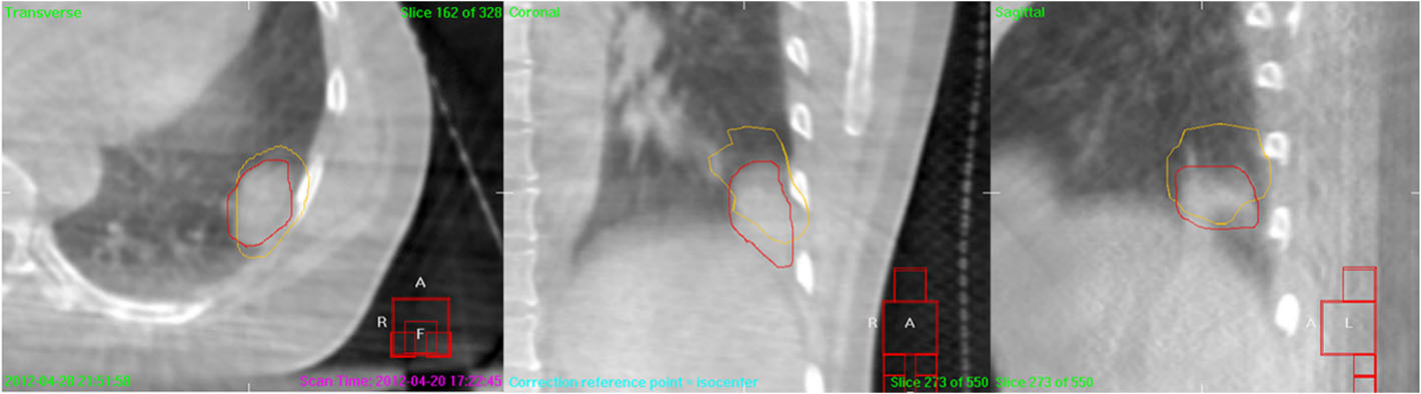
Precorrection cone-beam CT (CBCT) image for an protocol NSCLC patient in the thermoplastic mask. TP-3DCT GTV in *Yellow*; Precorrection GTV in *Red*
